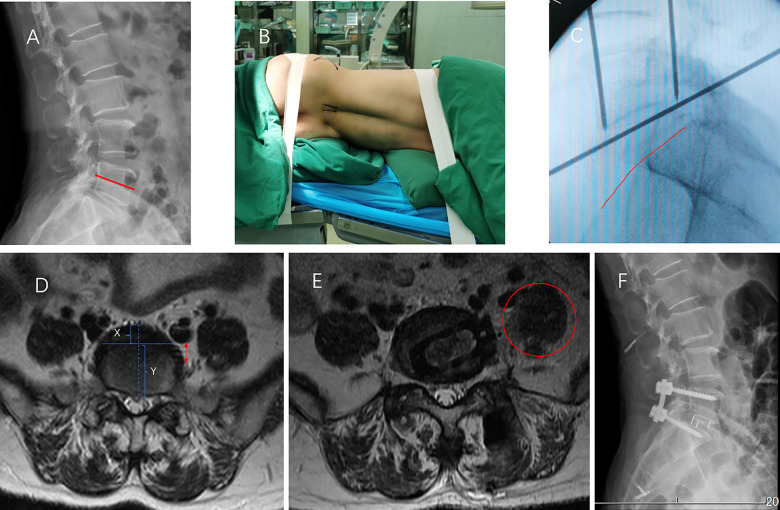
A 56-year-old woman underwent XLIF at the L5/S1 level. (A) Preoperative radiograph showed L5 degenerative spondylolytic spondylolisthesis, and the iliac crest was below the 1/2 line of the L5 vertebra (the red line). (B,C) The iliac crest (C red line) is lowered below the L5/S1 intervertebral space after folding the operating table (B). (D) The iliac vessels were located in the first quarter of the intervertebral space(X:Y < 1:3). There is no obvious nerve or vascular tissue between the psoas and the lateral side of the intervertebral space. (E) The red circle shows the working channel through the left psoas at L5/S1. (F) Radiographs taken at 3 days postoperatively showed a good cage position.
